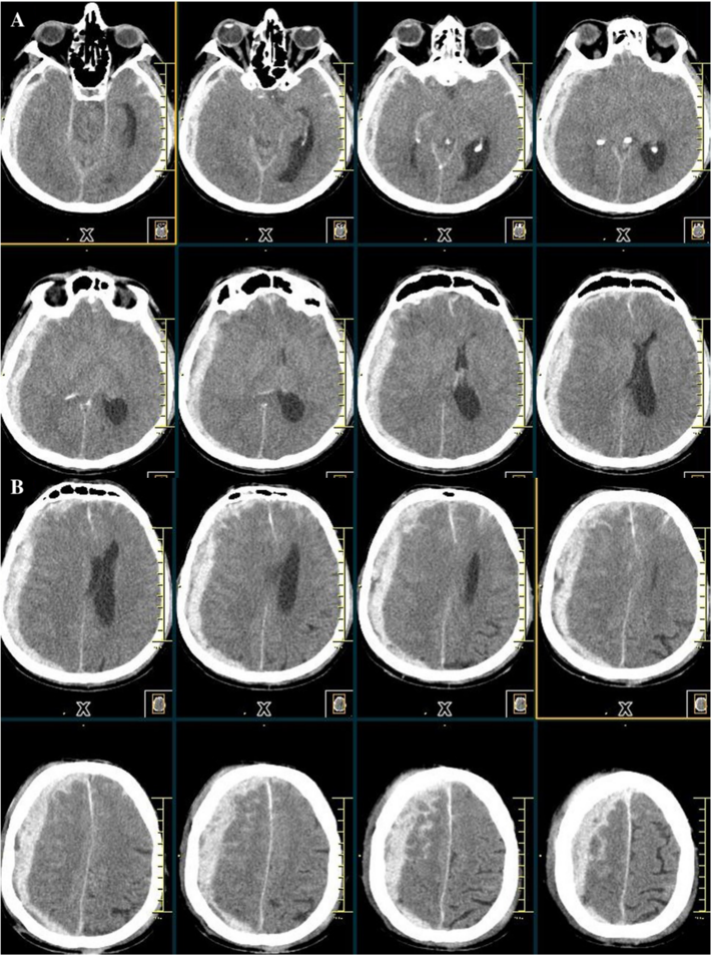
(A/B): Admission computed tomography showing right acute subdural hematoma.
